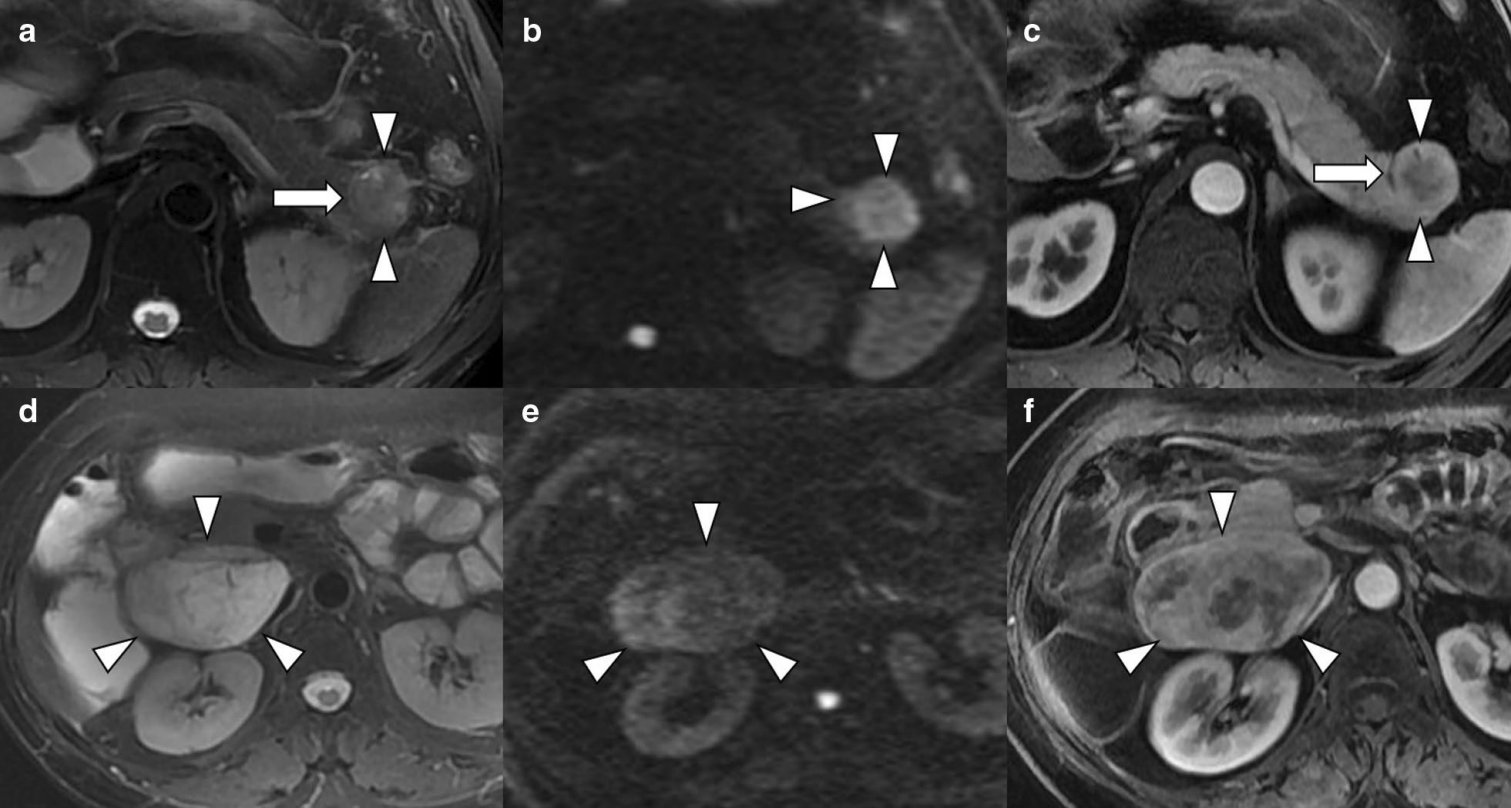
Pancreatic neuroendocrine tumors with regular tumor characteristics on T2WI (left), DWI (middle) and arterial phase (right) images. a–c a round, well-demarcated tumor with smooth contours is shown on MR images. The tumor-pancreas interface is sharp with pseudo-capsule (arrow). d–f MR images reveal oval but regular tumor located at the head of pancreas, the tumor-pancreas interface is clear and smooth. Although the pseudo-capsule is not demonstrated on MR images, the tumor is also categorized with regular shape
